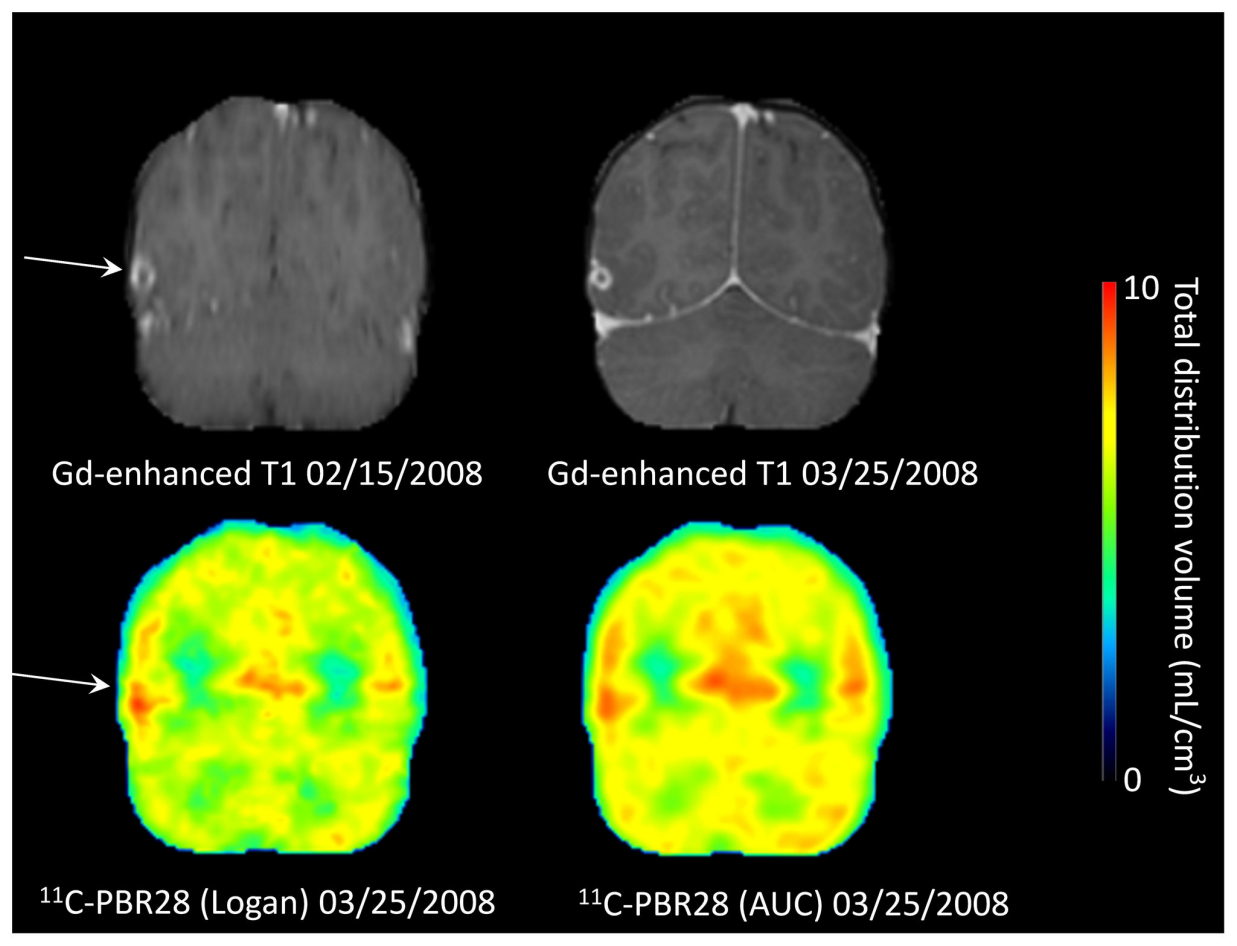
MR and PET images of patient 2 who showed a degenerating cyst (arrow). The patient presented with a seizure 85 days (01/06/2008) before the PET scan on 03/25/2008. On 02/15/2008 when the patient was asymptomatic, MRI scan showed a Gd-enhanced degenerating cyst in right temporal cortex (arrow). On the day of the PET scan (03/25/2008), the Gd-enhanced lesion slightly shrunk, and the PET scan showed increased ^11^C-PBR28 binding based on Logan plot (21%) and area under the curve (AUC, 17%). The color bar applies to only the PET image on the left side.
